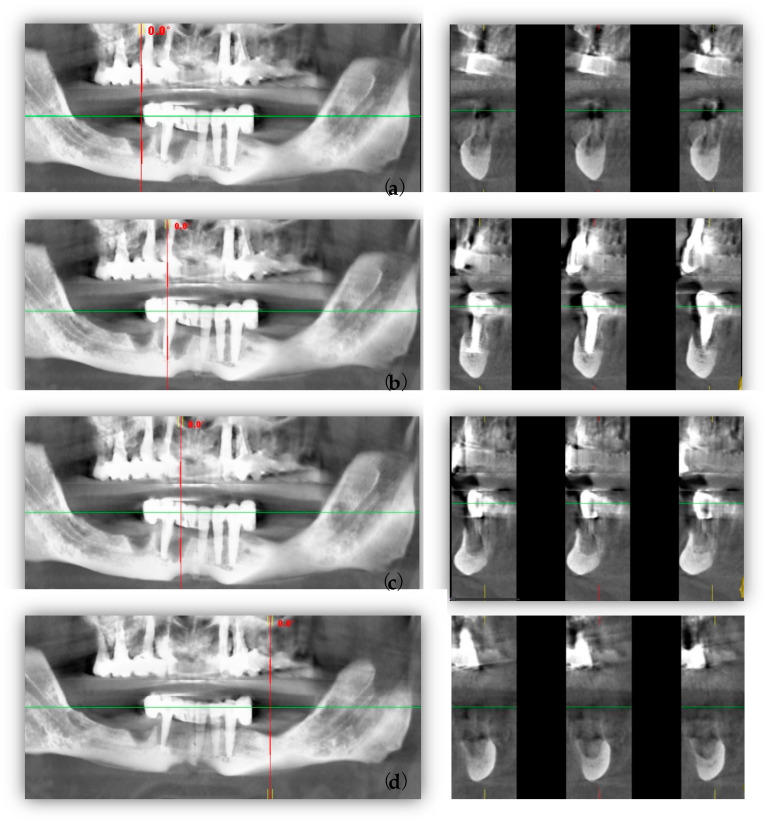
Pre-surgical CBCT images: (a) panoramic (green line) and cross-sectional (red line) views of bone defect after implant failure; (b) panoramic (green line) and cross-sectional (red line) views of 4.3 implant; (c) panoramic (green line) and cross-sectional (red line) views of bone defect after implant failure; (d) panoramic (green line) and cross-sectional (red line) views of bone defect after implant failure.
